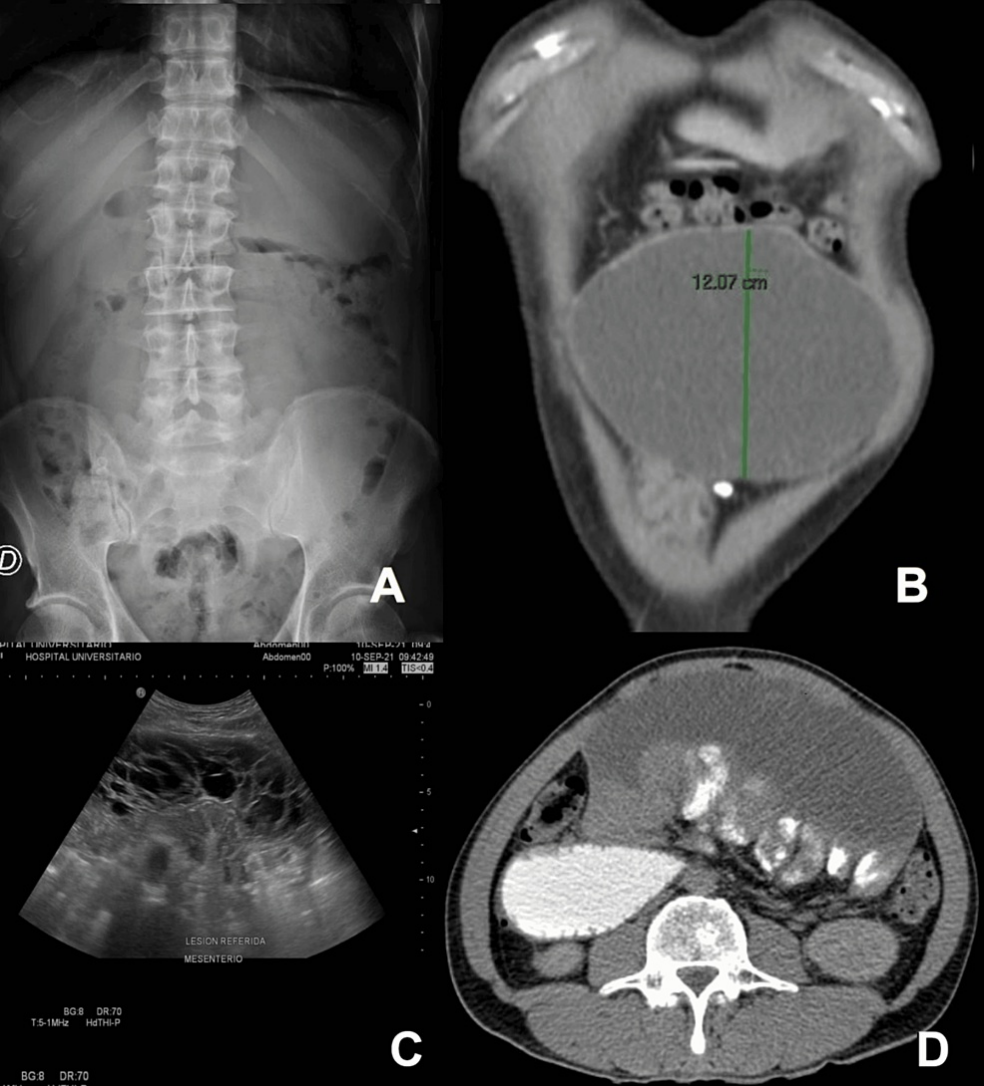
Imaging Findings of Mesenteric Mass A. Abdominal radiography reveals a non-calcified mesogastrial mass exerting pressure on abdominal structures. B. Abdominal ultrasound displaying irregular, well-defined, anechoic mesenteric lesions with multiple fine septae. C. Abdominal CT scan (coronal view) highlighting the mesenteric mass. D. Abdominal CT scan (axial view) detailing a well-defined, round, homogeneous, hypodense mass measuring approximately 15.4 × 12.07 × 7.8 cm, without contrast enhancement, equating to a volume of 756 mL. CT: computed tomography
